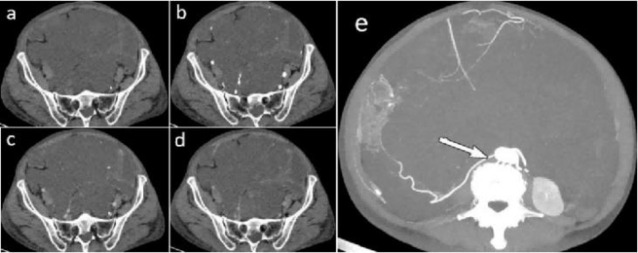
Axial plain (a) and contrast CT (b: arterial phase, c: venous phase, d: delayed phase) demonstrates multiple irregular low-density shadows in abdomen pelvic cavity, with arterial blood supply and local mild to moderate enhancement. The abdominal CT angiography (e) demonstrated that the tumor had an abundant blood supply
